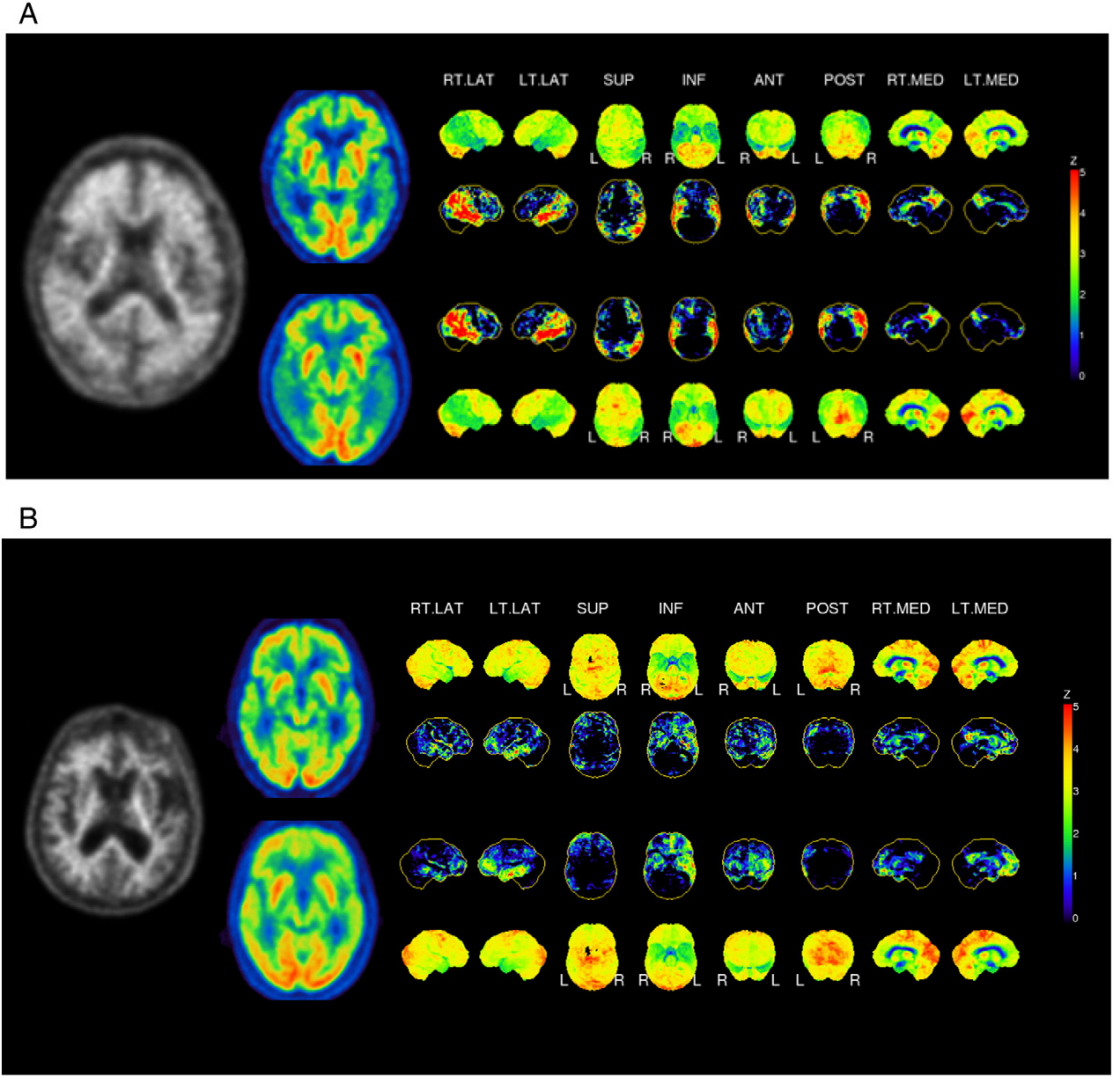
3D-SSP images of a 79 year old male person with clinical presentation of AD (A) and an 81 year old male person with clinical presentation of FTLD (B). Normalized count and *Z*-maps for FDG (upper row) and FBB_0–5_. The map depicts areas with less uptake compared to normal controls. R: right; L: left; PCC: posterior cingulate cortex
